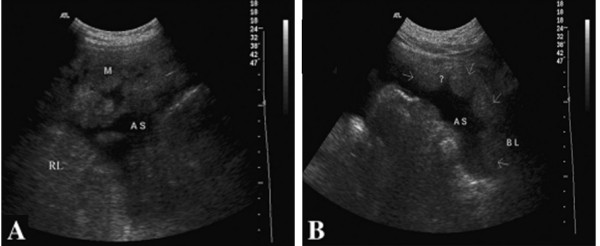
A 44-year-old man was hospitalized with abdominal distension. Ultrasonography showed cake- or nodosity-shaped thickening of the omentum (A) and peritoneum (B) with homogeneous hypoechoes. His pathological diagnosis was NHLL(Non-Hodgkin's lymphoma).
